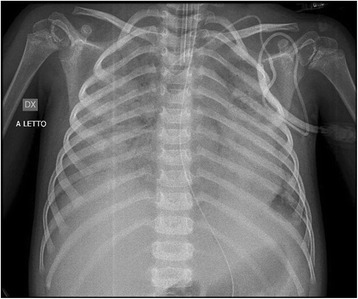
A chest X-ray scan showing bilateral diffuse acute distress respiratory syndrome at Intensive Care Unit admission
